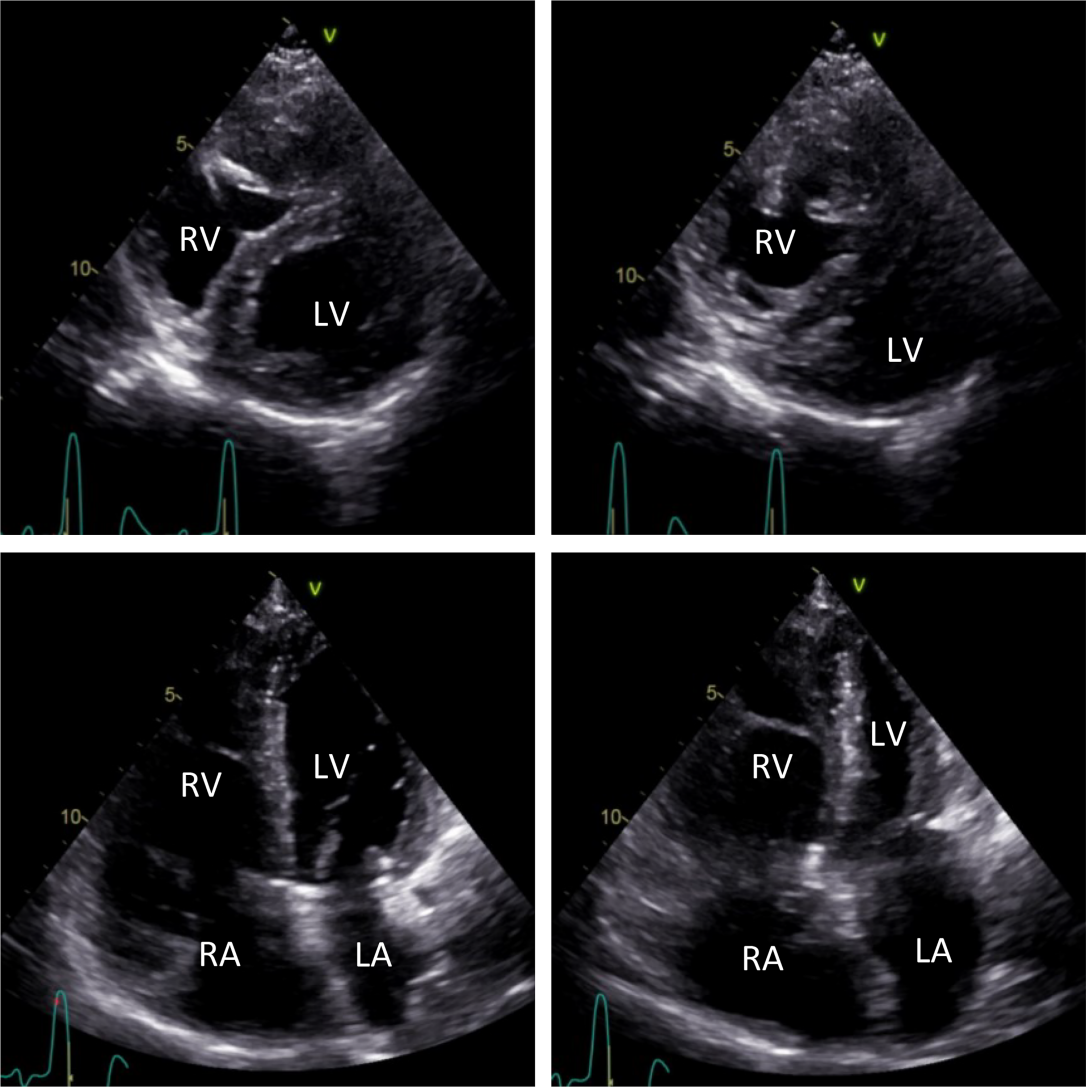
Transthoracic echocardiographic short-axis images (top) and long-axis four-chamber images (bottom). Echocardiographic short-axis views show a D-shaped, mildly hypertrophic LV during diastole (left) and systole (right). Mild dilation of the RA and RV can be observed in echocardiographic long-axis four-chamber views during diastole (left) and systole (right). LV, left ventricle; LA, left atrium; RA, right atrium; RV, right ventricle.
